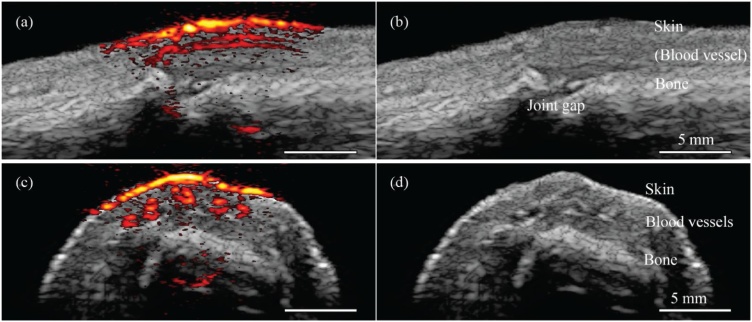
Co-registered photoacoustic and ultrasound images of a human proximal interphalangeal joint in sagittal (a) and transverse (c) view. (b) and (d) are anatomical-only ultrasound images from (a) and (c), respectively. Reprinted from Ref. [51].
